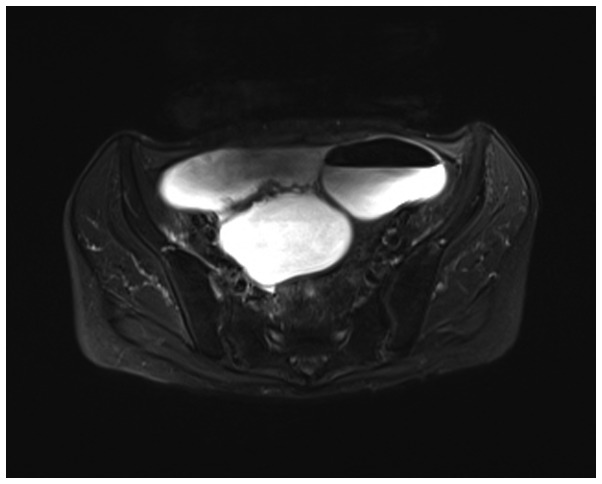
Magnetic resonance imaging of pelvic cavity revealing the lower intestinal obstruction.
